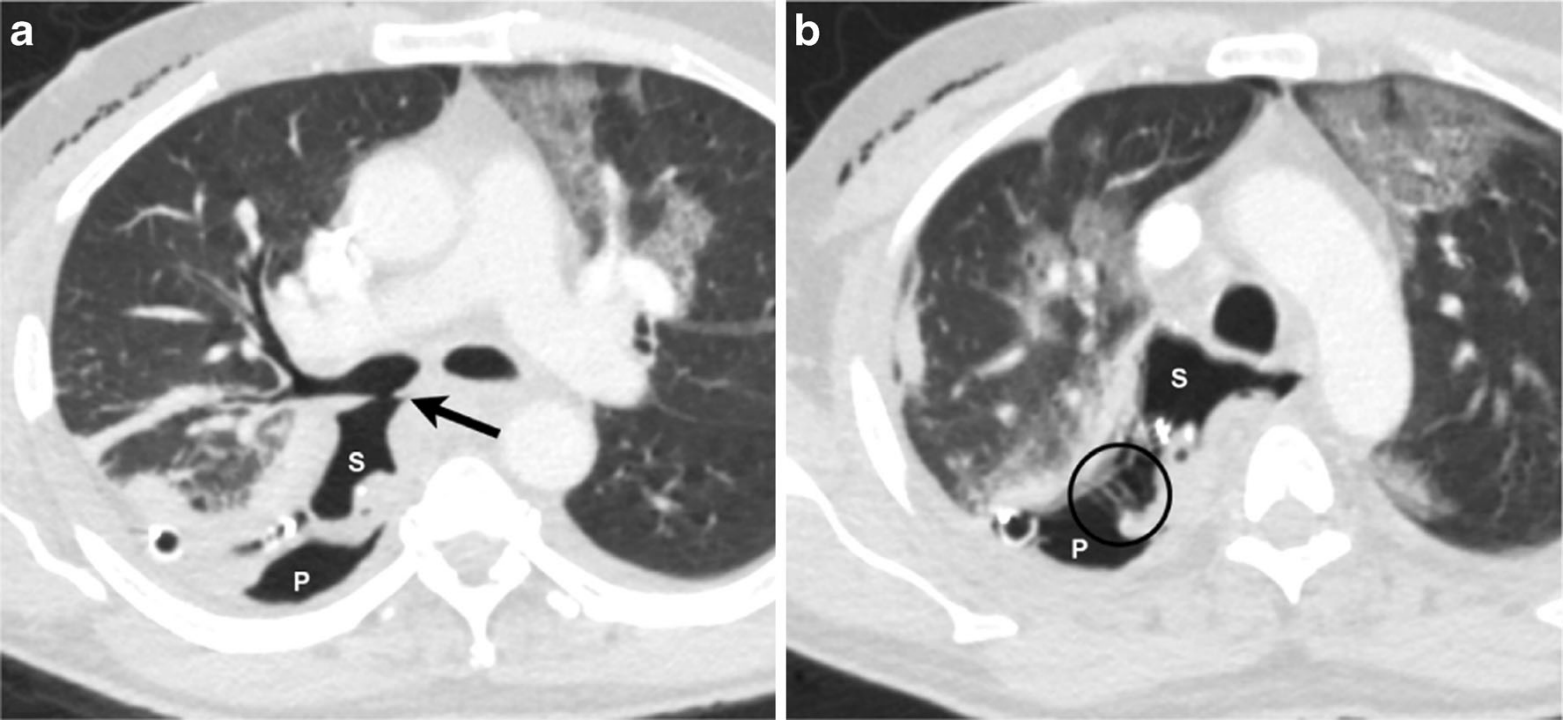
A 47-year-old male, post gastric pull through for oesophageal cancer. Axial CT (a) image through the mid-chest demonstrates direct communication of the right main stem bronchus with the stomach (S) (*black arrow*) resulting in a broncho-gastric fistula. Several slices inferiorly (b), posterior gastric wall is dehiscent (*circle*) and communicates with the pleural space (P), resulting in a gastropleural fistula. Note the chest tube within the posterior right pleural space
